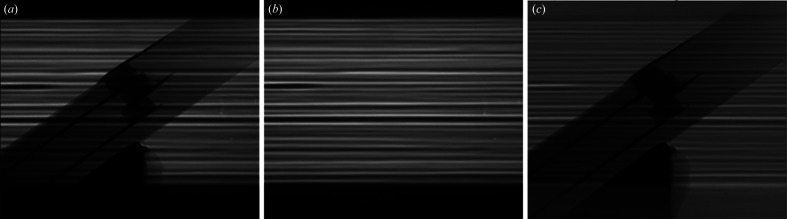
Radiographic images obtained at BAMline using 10× magnification (0.72 µm pixel size), showing the unnormalized image of (*a*) a 0.5 mm-thick LTCC test device, (*b*) a flat-field image and (*c*) the flat-field corrected image.
